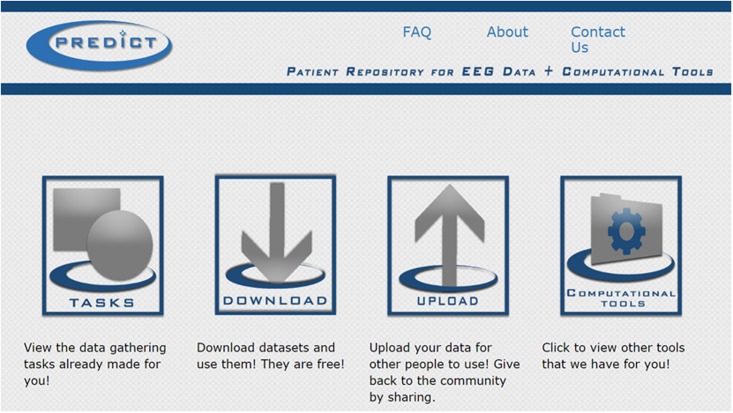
Screenshot of the PRED+CT home screen (www.predictsite.com).
